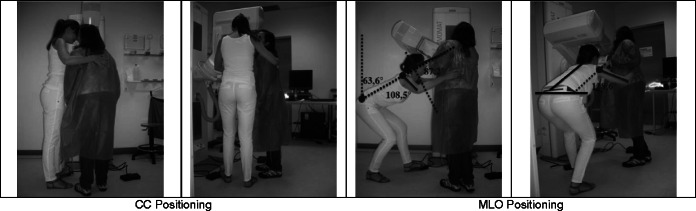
Postures assumed by a radiographer taller than the patient
